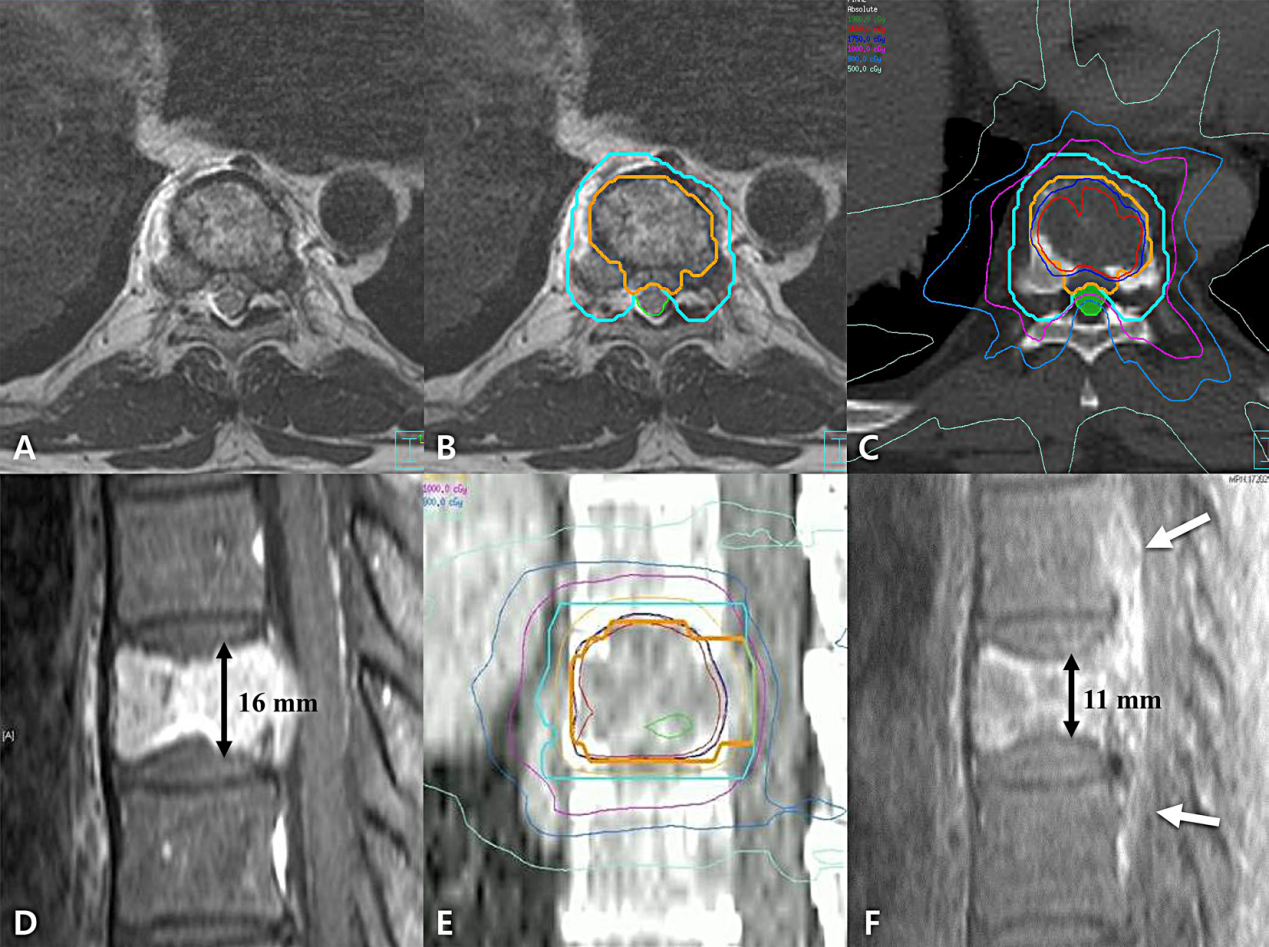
(A) Axial view of planning magnetic resonance image (MRI), (B) example of target delineation in axial view, (C) example of dose distribution in axial view, (D) sagittal view of planning MRI, (E) example of target delineation and dose distribution in sagittal view, (F) sagittal view of follow-up MRI at 3 months after stereotactic ablative body radiotherapy (SABR). The patient, who was 60-year-old male with spinal metastasis with radiographic grade of cord compression III, received SABR of 18 Gy in 1 fraction. Gross tumor volume (GTV), clinical target volume, and spinal cord was delineated in orange, sky blue, and green color, respectively. Because the GTV contacts with spinal cord, we concerned the spinal cord delineation more than GTV delineation. The GTV and spinal cord volume are exclusive to each other (B). The spinal cord dose constraint was also more concerned rather than GTV dose for spinal cord saving (C). Before SABR, we observed the tumor infiltration along the posterior longitudinal ligament (PLL) (D). The tumor progression was shown in both cranial and caudal directions along the PLL at 3 months after SABR (white arrow; F). The patient had pre-existing vertebral compression fracture (VCF) with Spinal Instability Neoplatic Score of 8 (D). There is decrease in height of vertebra body from 16 mm to 11 mm at 3 months after SABR which means the progression of pre-existing VCF (F).
